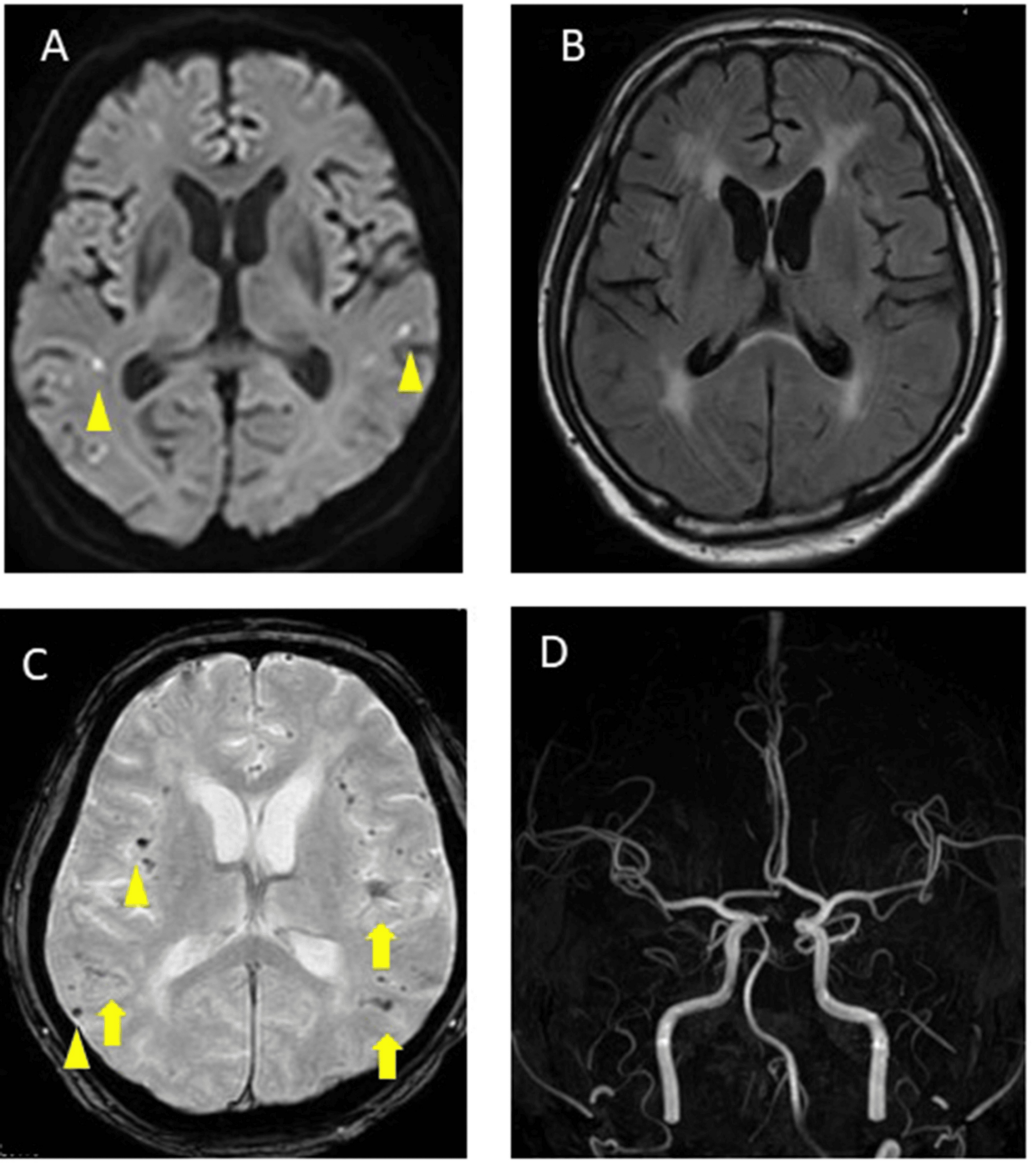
Brain magnetic resonance imaging and magnetic resonance angiography (MRA) on day 29 A: Diffusion-weighted imaging (DWI) showed hyperintensities in both parietal regions (yellow triangle). B: Fluid-attenuated inversion recovery image (FLAIR) showed no change in white matter lesions. C: T2* imaging demonstrated cortical superficial siderosis in the left frontal and both parietal sulci (yellow arrow). D: MRA showed no stenosis or occlusion in the major arteries.
